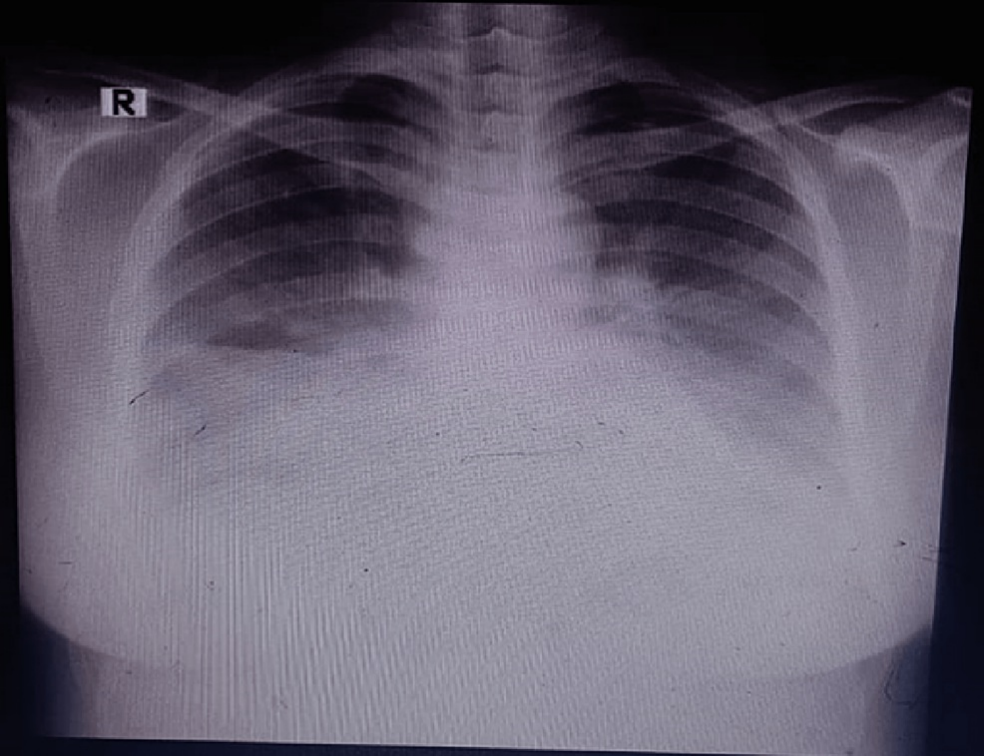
Initial chest x-ray on October 15, 2022, which showed pleural effusion and pericardial effusion.
